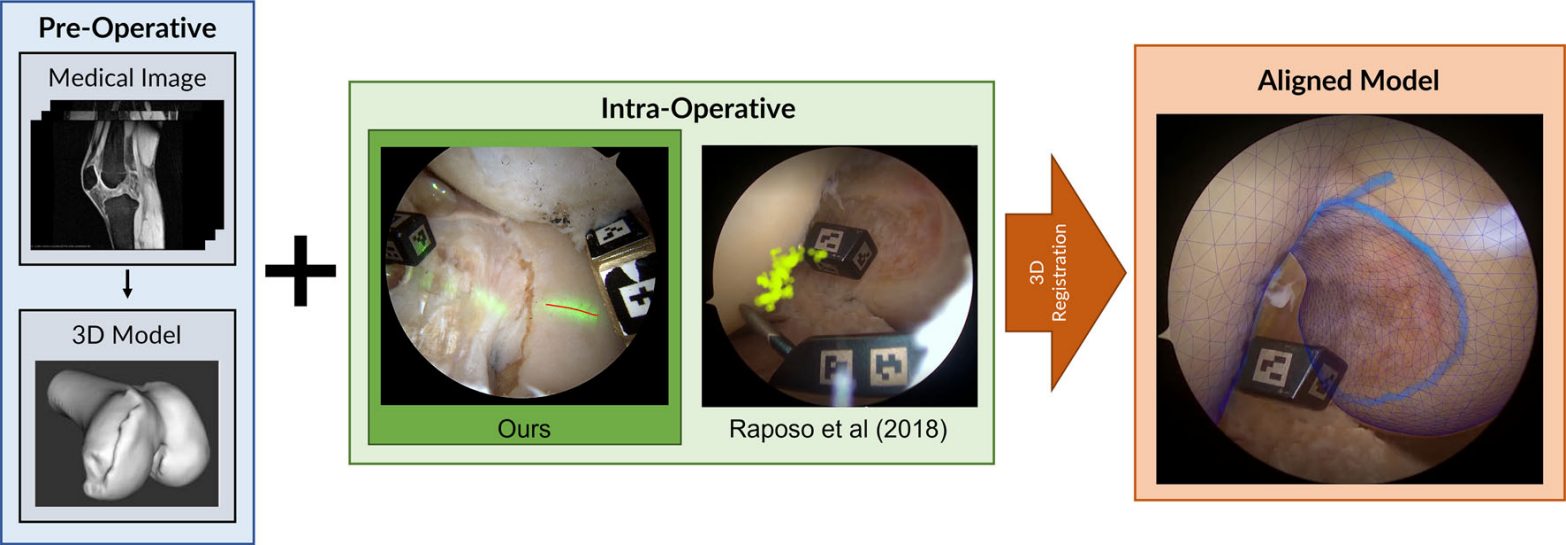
The existing solution for VBSN uses information from preoperative imaging, such as MRI, and requires the surgeon to digitize the surface of the bone with a touch probe, enabling the 3D reconstruction of points on bone surface [16]. This paper proposes to replace the use of a touch probe by an off-the-shelf laser scanner to reconstruct the points. After 3D registration is completed, the preoperative model can be overlaid with the anatomy using augmented reality. For better assessment of the registration quality, the preoperative model (shown as a mesh grid on the right) is augmented with a blue contour representing the intercondylar arc
